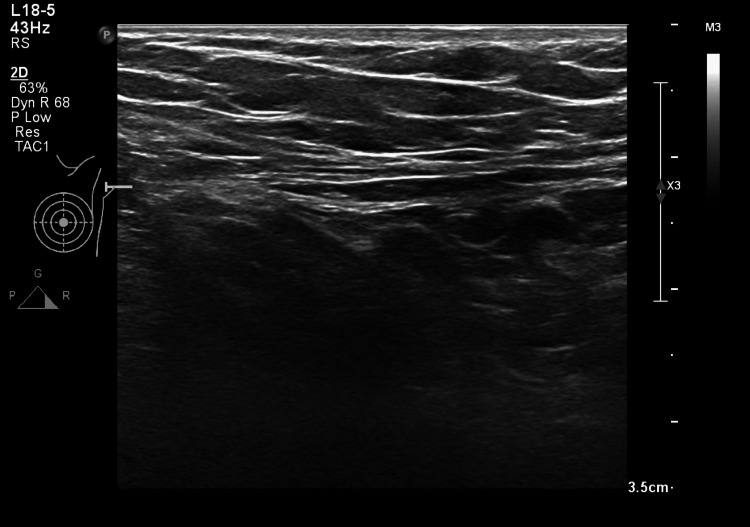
Ultrasound image of the axilla which shows no pathology.
